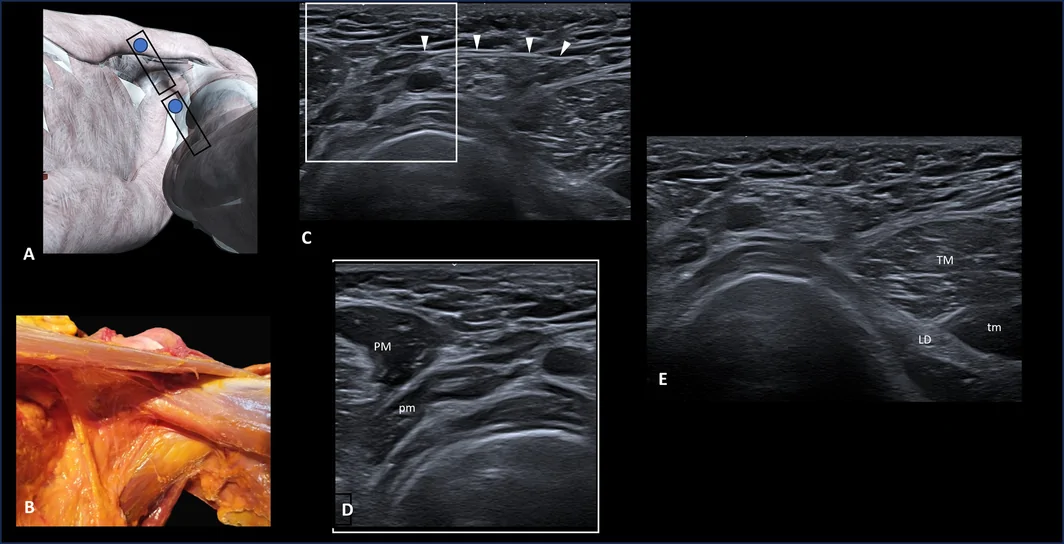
Axillary fascia. A, Probe positioning. B, Anatomical dissection showing the axillary fascia forming the floor of the axilla, extending between the pectoral and latissimus dorsi margins. Note its continuity with the brachial and clavipectoral fasciae and the presence of neurovascular perforations. C–E, Ultrasound images revealing the axillary fascia (arrowheads) as a thin, hyperechoic band separating the subcutaneous tissue from the deeper axillary contents, with adjacent visualization of lymph nodes and vascular elements. PM, pectoralis major muscle; pm, pectoralis minor muscle; TM, teres major muscle; tm, teres minor muscle; LD, latissimus dorsi muscle.
